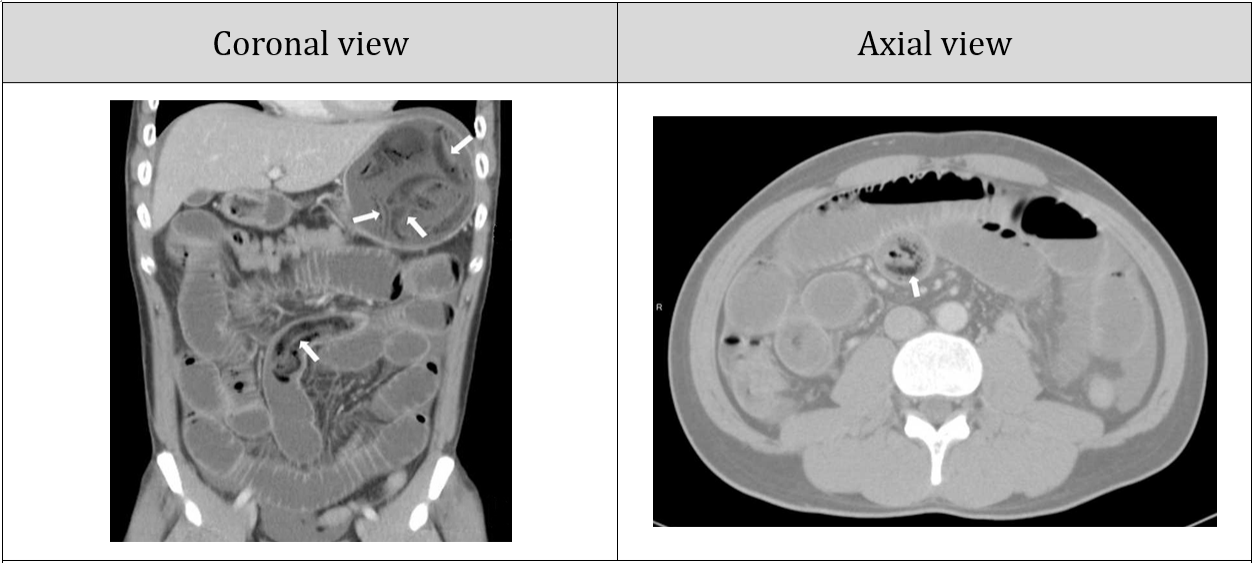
Patient’s abdominal computed tomography scan
